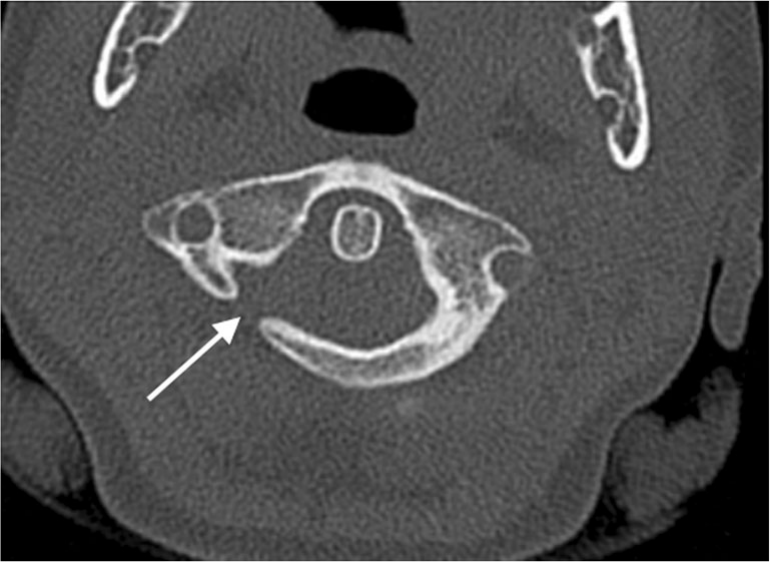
Unilateral posterolateral rachischisis: axial CT image through the atlas (C1 vertebra) of the craniocervical junction of an 8-year-old female child involved in a road traffic accident (pedestrian versus car) who sustained head injuries with seizures. A developmental unilateral right posterolateral rachischisis is present (*arrow*). There were no craniocervical junction or sub-axial cervical spine fractures present
